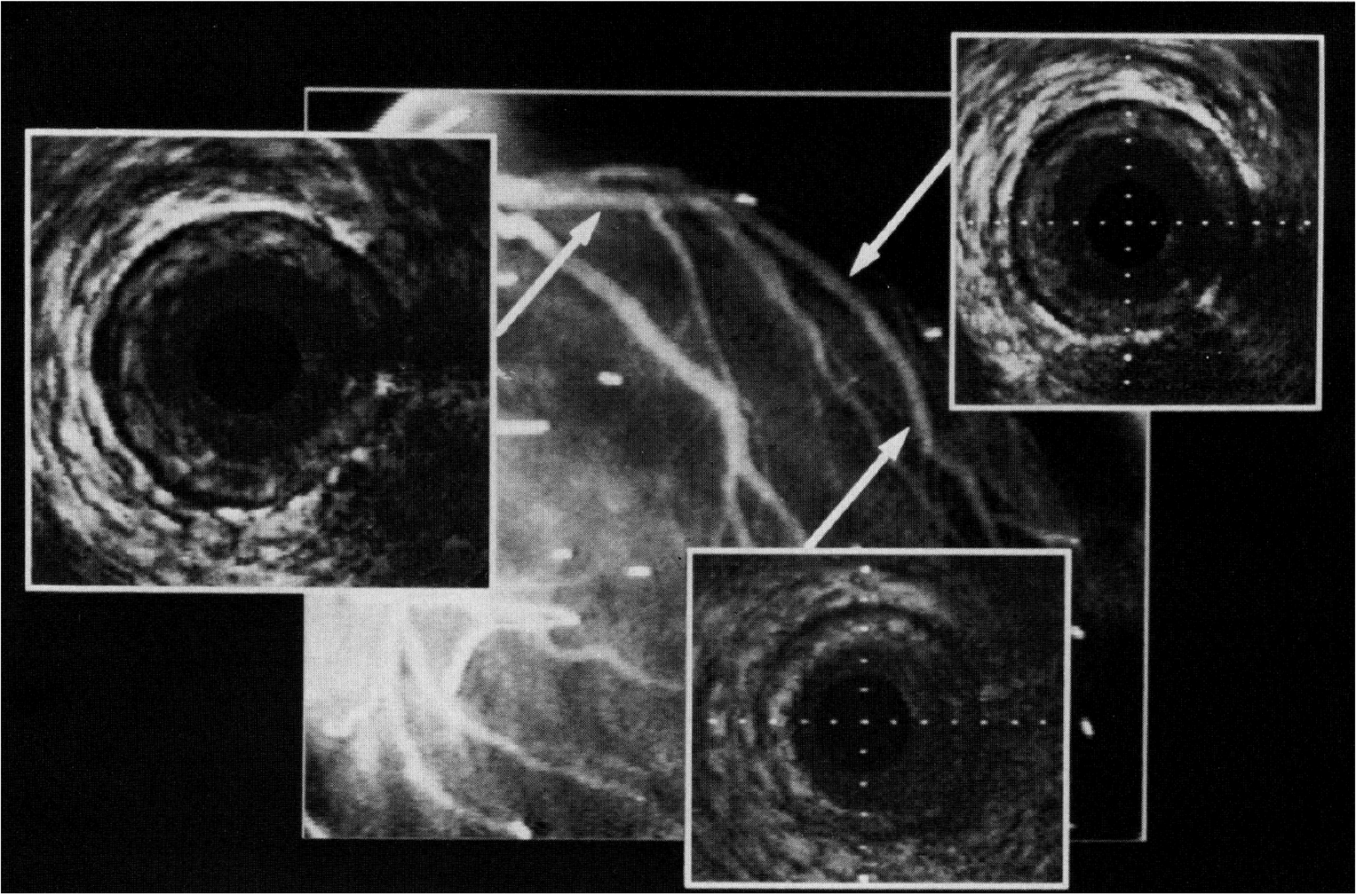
Invasive coronary angiogram with intravascular ultrasound images from three representative sites in the left anterior descending coronary artery (LAD) of a 37-year-old man 3 years after heart transplantation. Although the angiogram is without evidence of coronary disease, the ultrasound images demonstrate severe concentric intimal thickening throughout the proximal and mid LAD. Used with permission from St. Goar et al. Circulation. 1992 Mar;85 [3]:979–87
